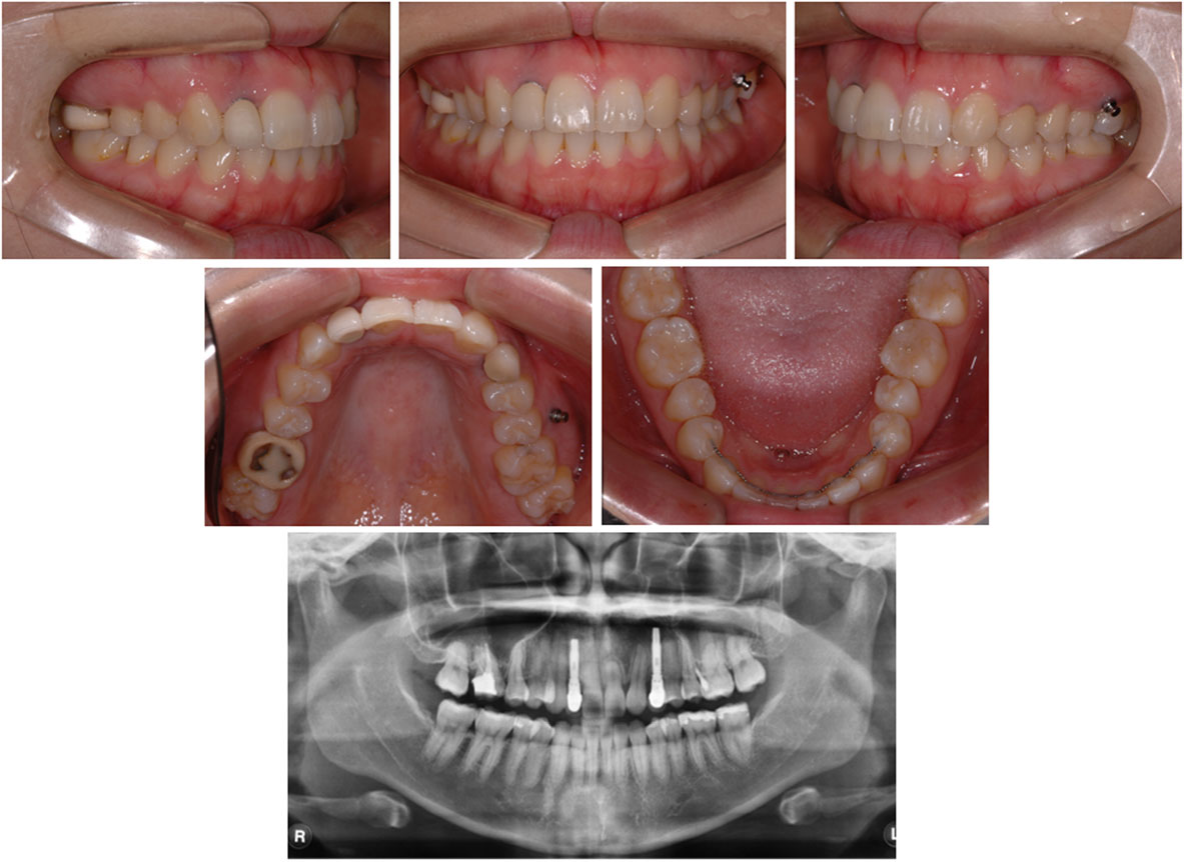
Post-treatment intraoral photographs and panoramic radiograph after implant restoration
